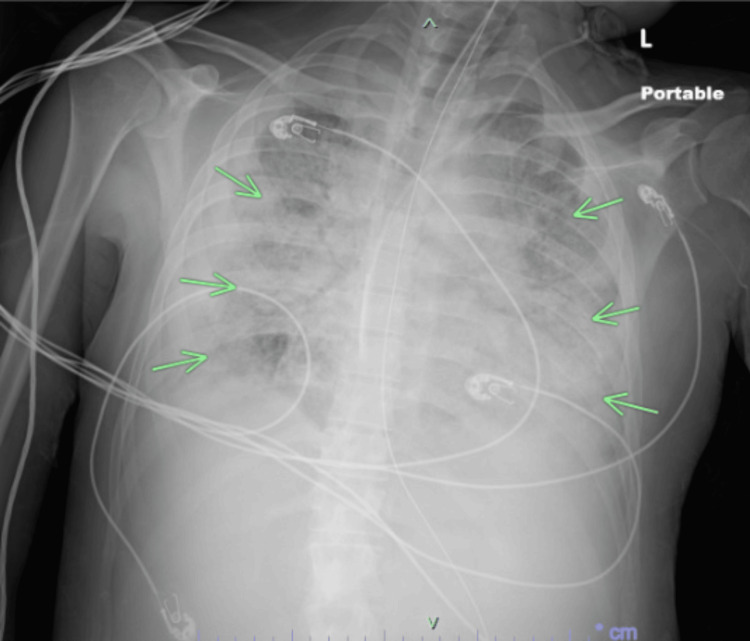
Chest X-ray post-intubation that showed significant worsening of bilateral infiltrative disease (as emphasized by the arrows), indicative of acute hypoxic respiratory distress.
